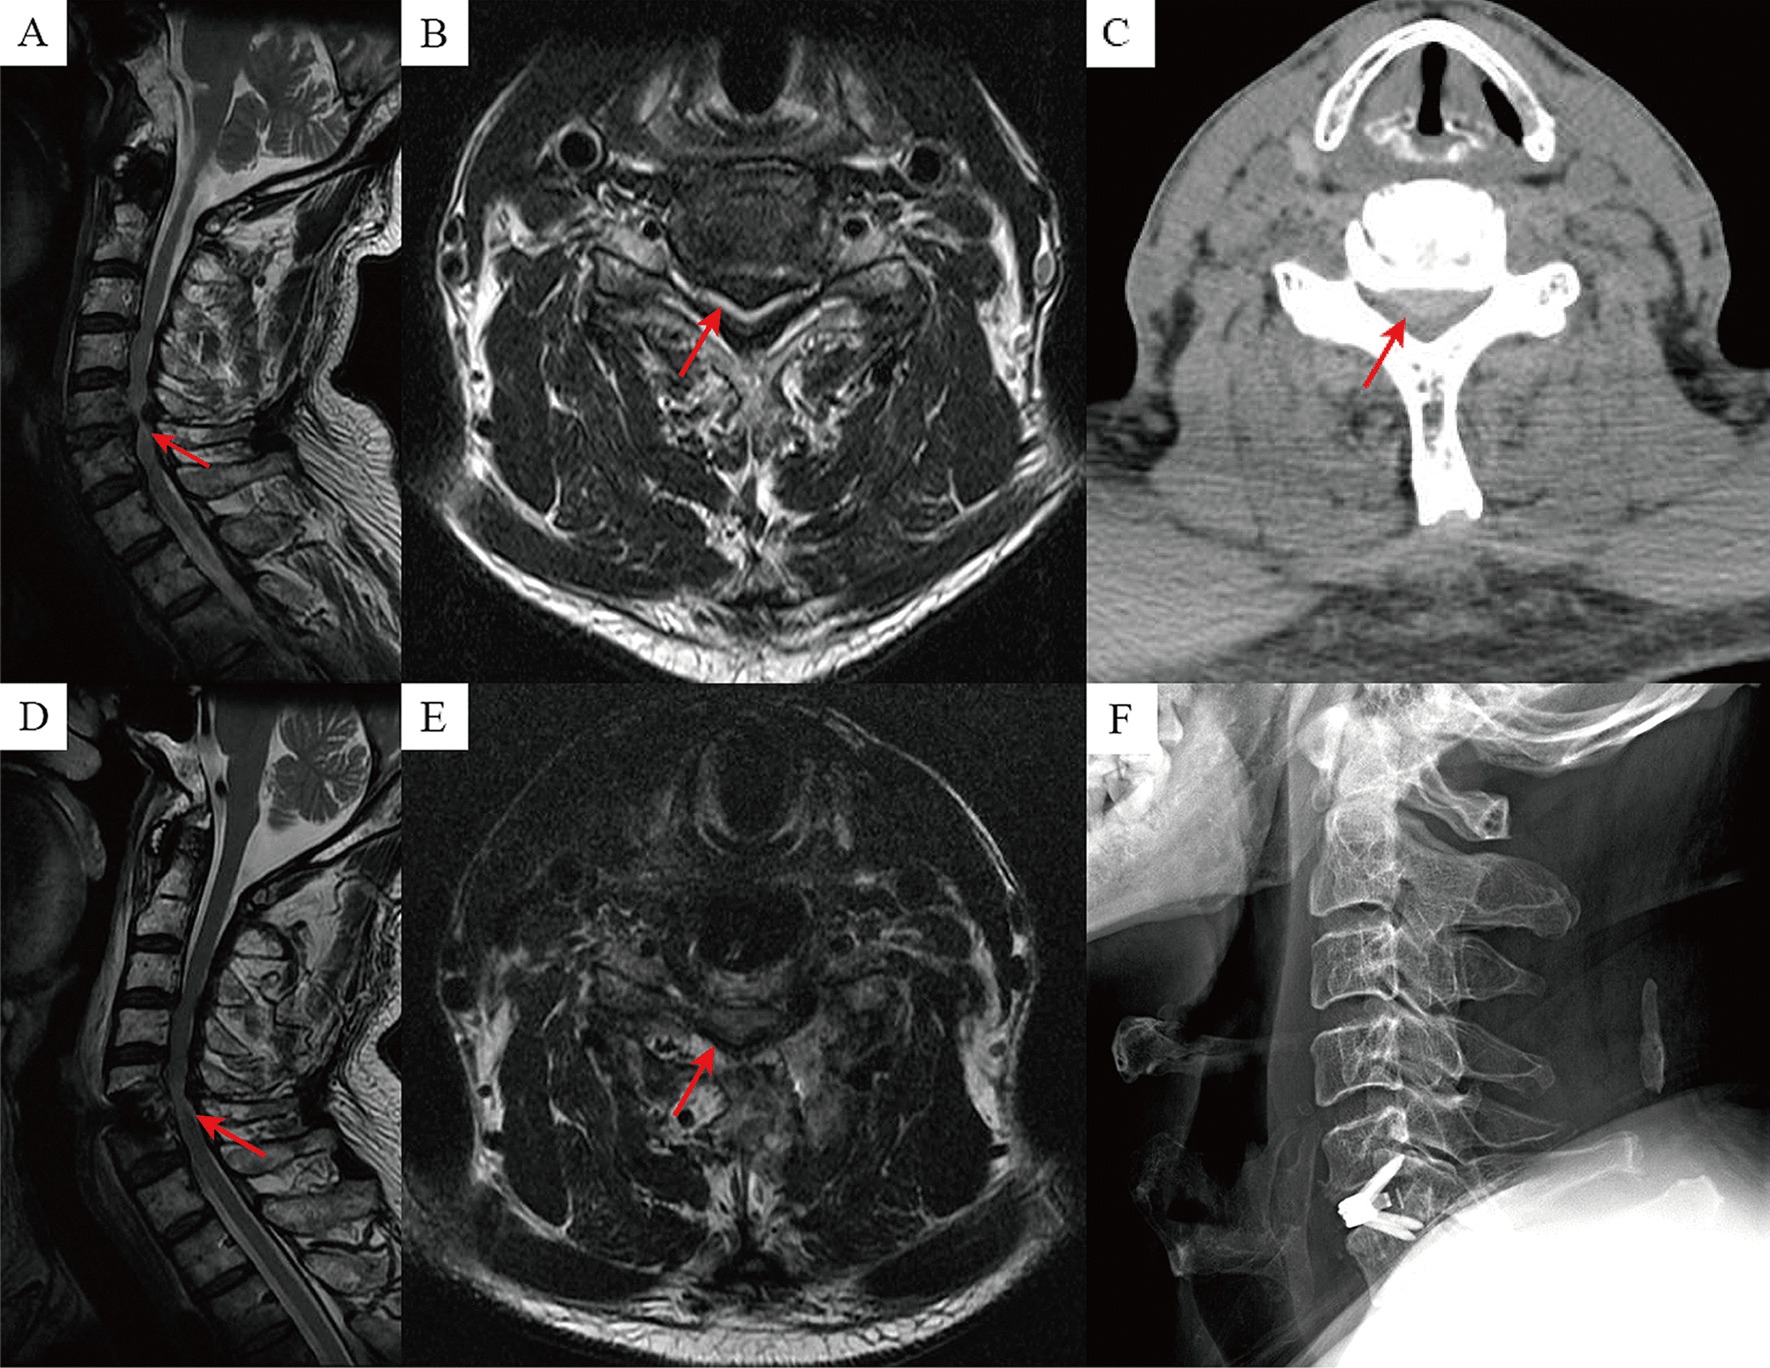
Images from a patient treated with ACDF. A–C Preoperative MRI and CT showed spinal stenosis of C5–6 segment, and compression of the spinal cord; D, E Postoperative MRI showed adequate decompression of the spinal canal and spinal cord; F Postoperative interbody fusion with internal fixation
